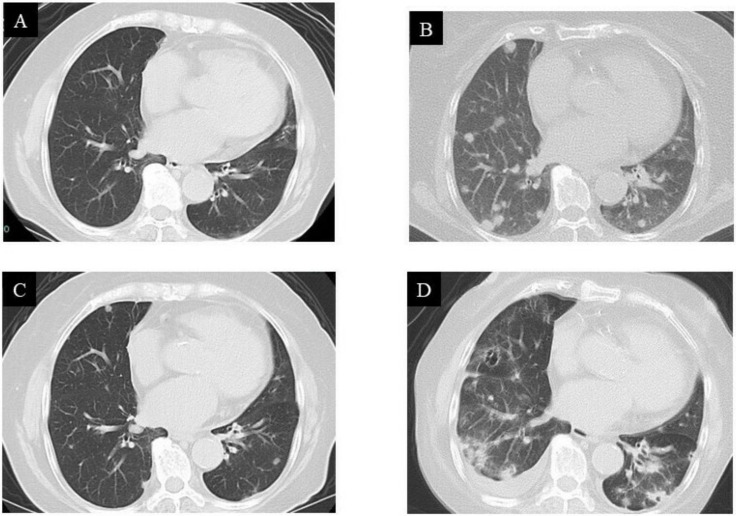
Computed tomography (CT) images of the lung: (A) no pulmonary metastases observed before treatment; (B) multiple pulmonary metastases observed three months after surgical resection; (C) metastases rapidly regressed after pazopanib treatment; (D) metastases recurred 11 months after pazopanib treatment.
